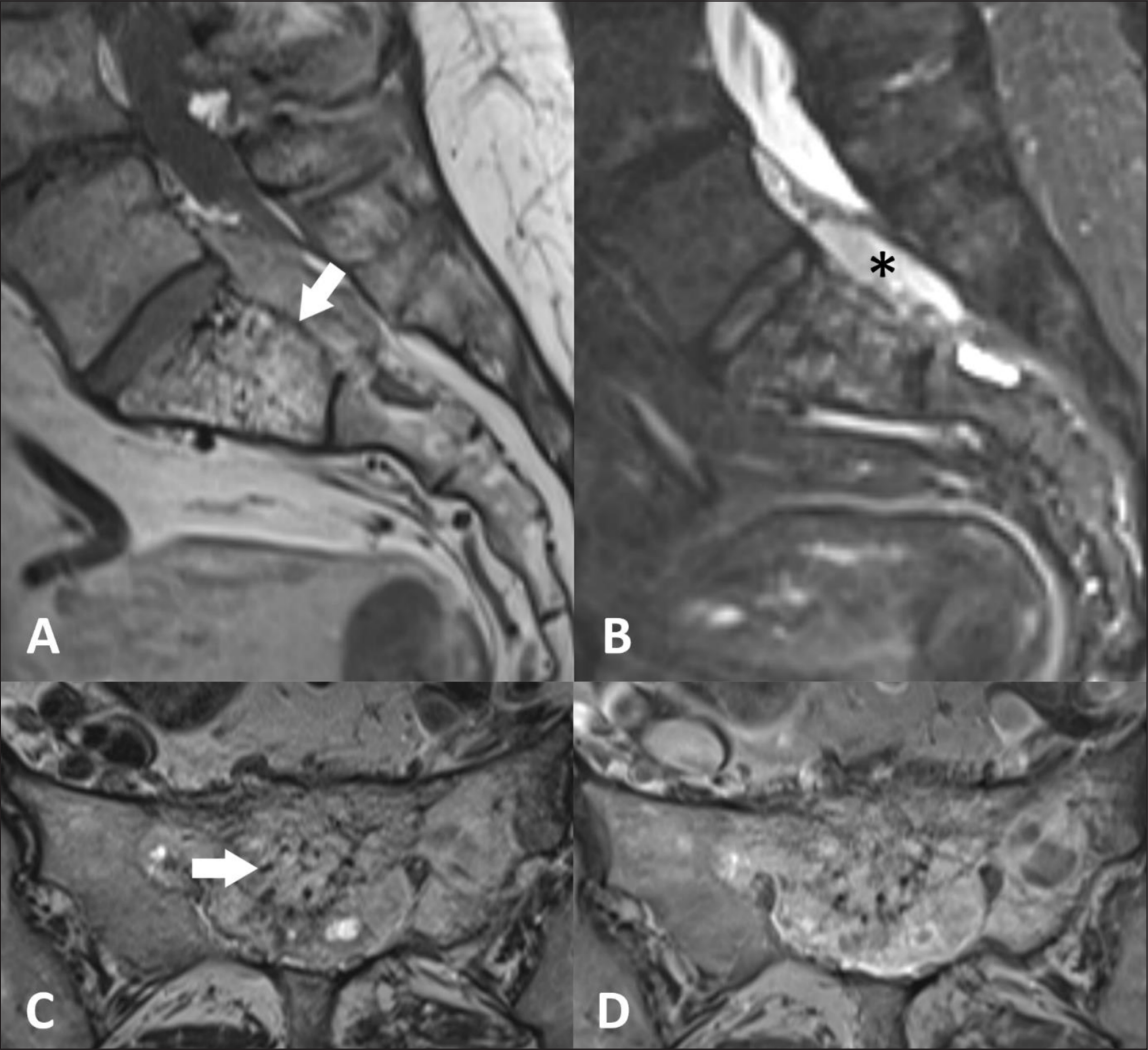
A 65-year-old female with sacral hemangioma. (a) Sagittal T1-weighted MR image and (b) sagittal fat saturated T2-weighted MR image shows a S1 sacral hemangioma with an atypical extra-osseous component extending into the spinal canal (asterix). Note the maintained vertebral height and cortical integrity (arrow). (c) Axial T2-weighted MR image shows hyperintense signal with multiple punctuate hypointense areas (polka-dot sign) (arrow). (d) Axial post-contrast T1-weighted MR image shows heterogeneous enhancement of the tumour.
